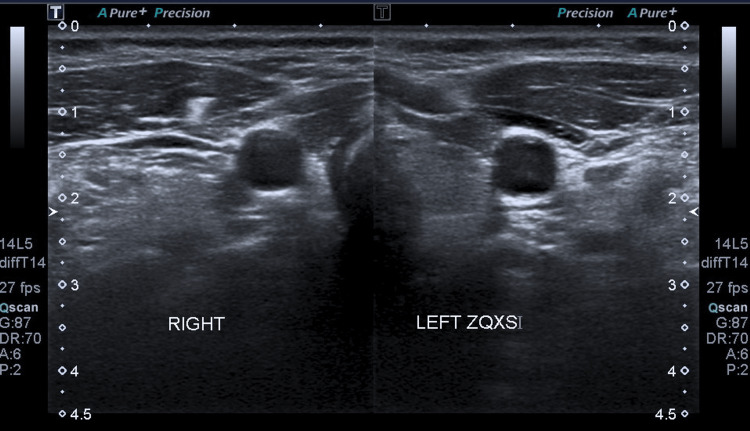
Neck ultrasonography showing perivascular hyperechogenicity on the left common carotid artery.
